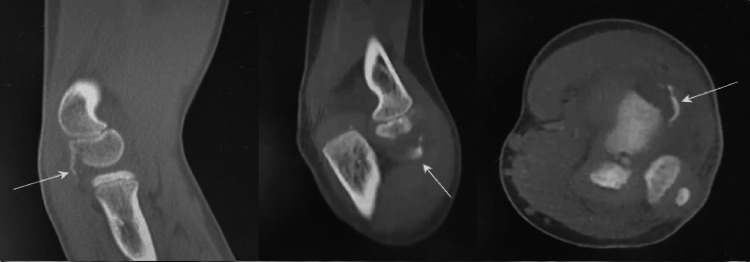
CT scan From left to right: lateral view, posteroanterior view, and axial view showing a Kocher-Lorenz fracture with posterolateral displacement (white arrows).
